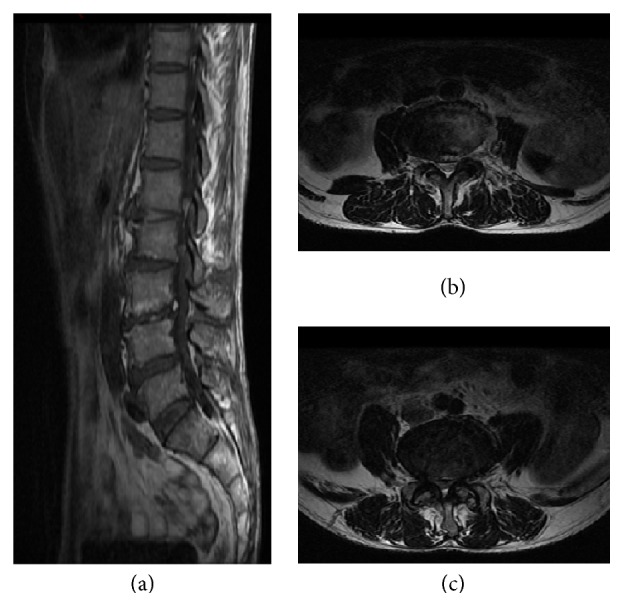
Both the T1 sagittal (a) and coronal MRI images of L3-4 (b) and L4-5 (c) suggested that the herniation and lumbar stenosis compressed the thecal sac at both levels, and lumbar degenerative changes were also reported.
